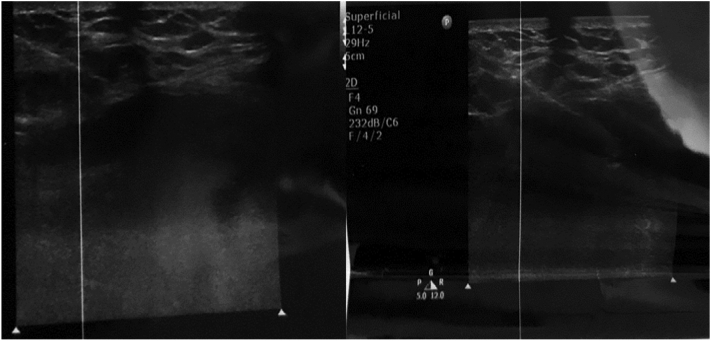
Images of ultrasound guided percutaneous biopsy of right iliac bone mass.
